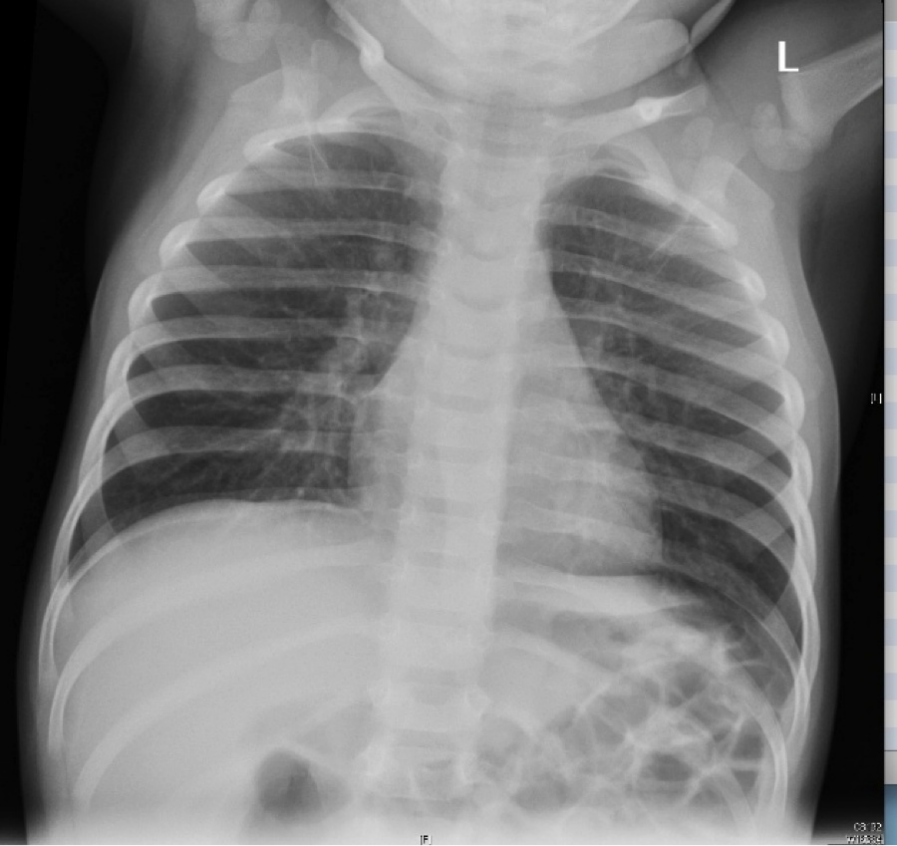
Chest X-Ray to be provided to trainee on request
